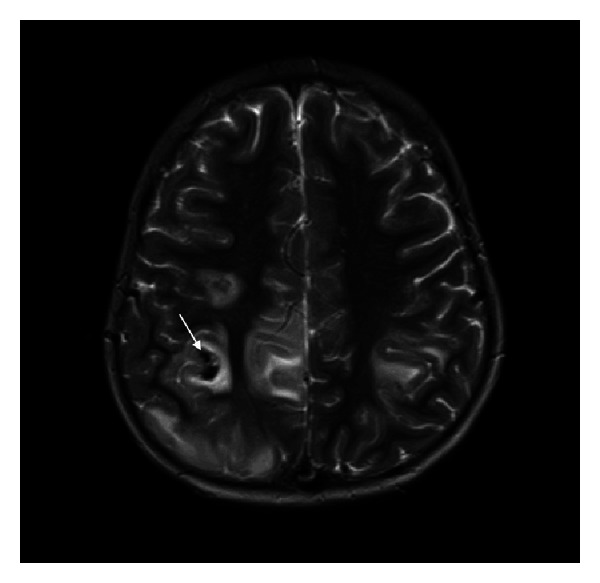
Axial T2-weighted MRI shows bilateral acute venous infarctions with hemorrhage (arrow) involving right frontal lobe.
